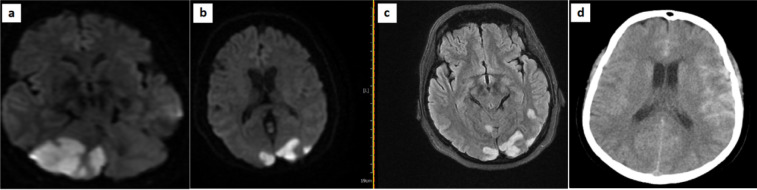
(a, b, c) Acute infarct in posterior cerebral artery (PCA) territory with restriction on diffusion-weighted magnetic resonance imaging (DWI) in a 53-year-old female with COVID-19 on the ninth day of admission; (d) hyper dense materials in brain sulci more prominent on left peritoneal lobe in favor of Subarachnoid hemorrhage (SAH)
